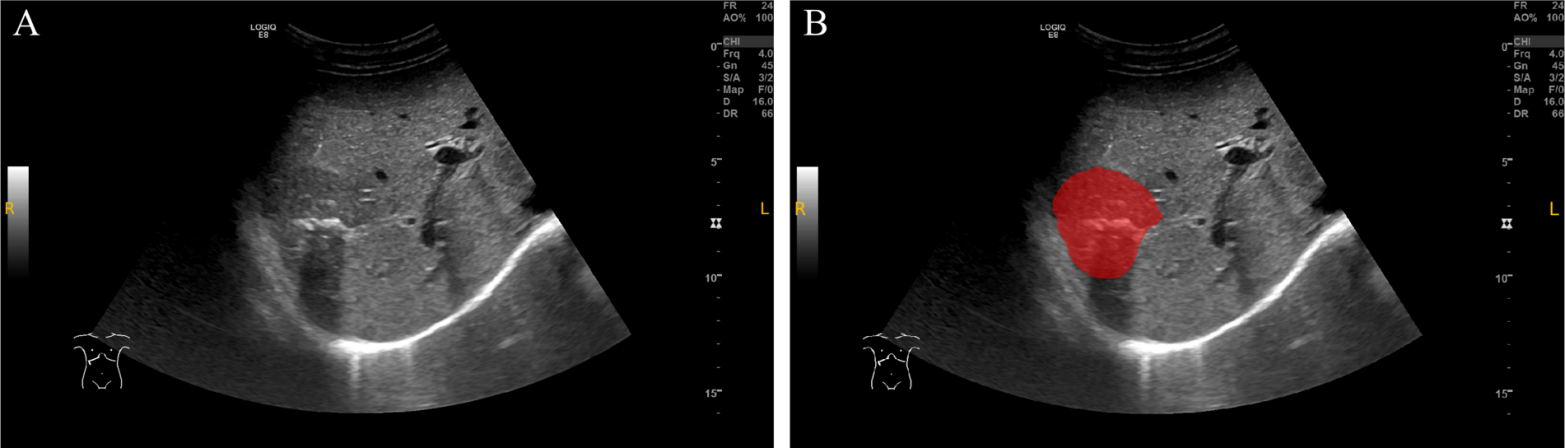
ROI delineation on ultrasound images. (A) Original grayscale ultrasound image of a patient with intrahepatic cholangiocarcinoma (ICC) combined with bile duct stones. (B) The region of interest (ROI) was manually delineated (red area) along the tumor margin. This case demonstrates the challenge in differentiating ICC with bile duct stones from intrahepatic bile duct stones with cholangitis (IBDS-IL) based on imaging alone.
